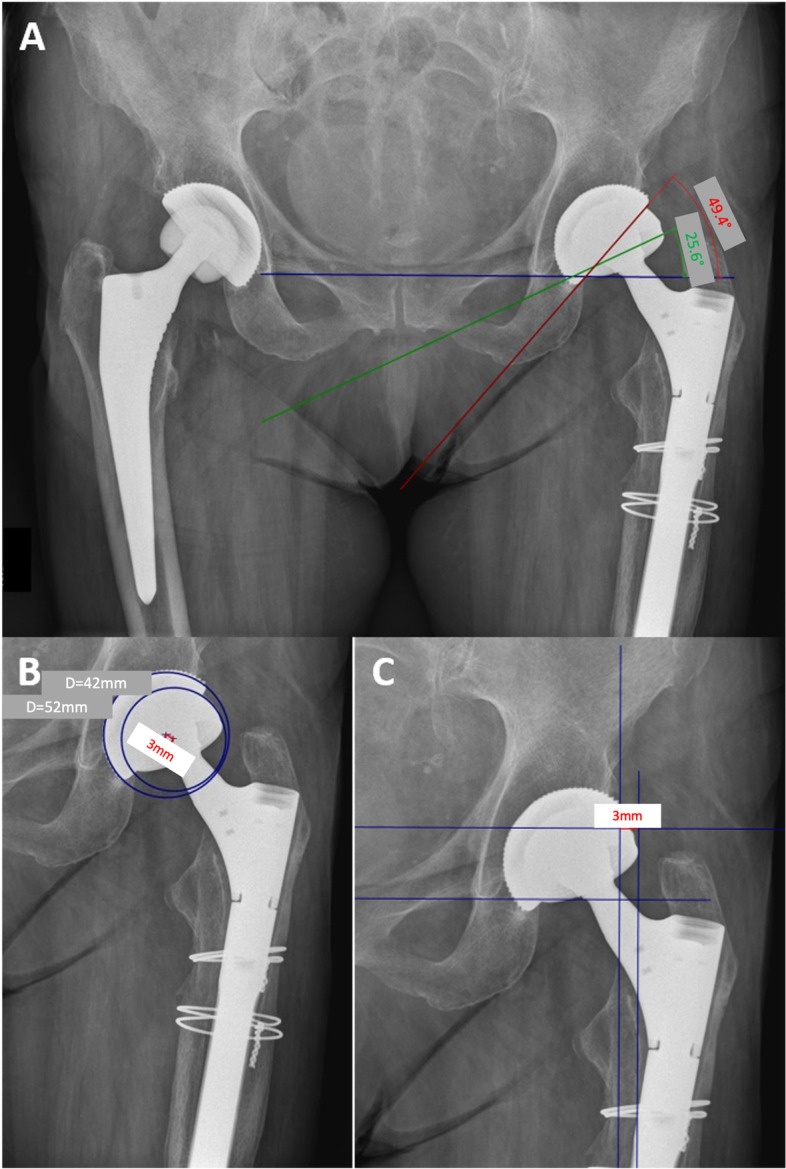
Radiographic measurements. (A) Measurement of inclination between well fixed cup (red line) and dual mobility cup (DMC; green line). The reference line is the connecting line between the two teardrops (blue line). (B) Measurement of the center of rotation distance (red connecting line) between the well-fixed cup and the DMC. (C) Measurement of the lateral edge distance between well-fixed cup and DMC (red line). Again, the connecting line between the lower aspects of Kohler’s teardrop figure (lower horizontal line) serves as the reference line
